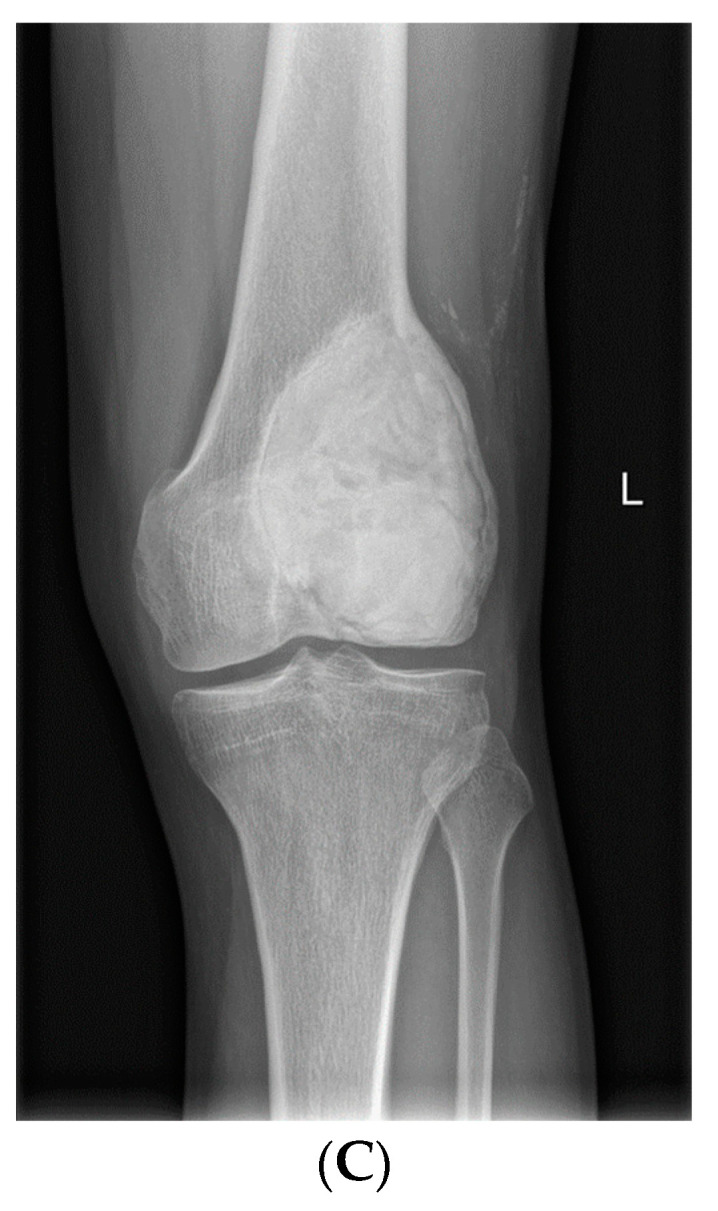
GCTB of the distal femur (X-rays). (A) Before denosumab therapy, (B) after 6 doses of denosumab, and (C) after intralesional curettage and PMMA cementoplasty.
